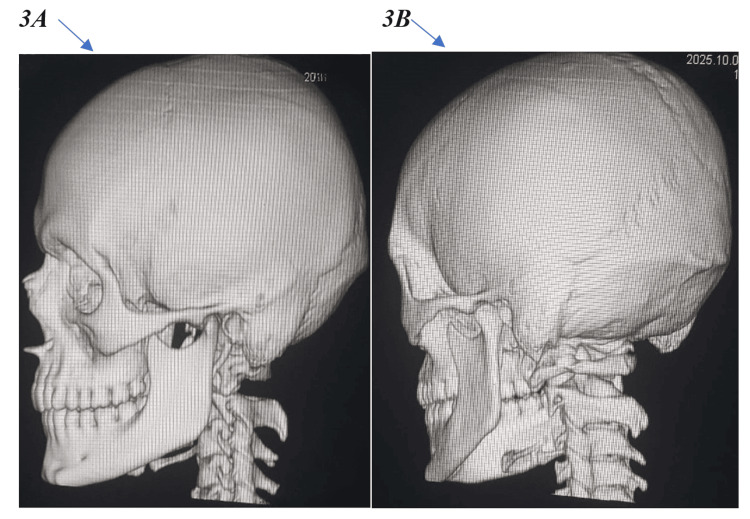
(A, B) Three-dimensional computed tomography (CT) reconstructions of the skull (lateral views) providing a comprehensive visualization of the calvarium. These images confirm the absence of any visible fracture or depression of the outer table, highlighting the isolated nature of the inner table injury.
